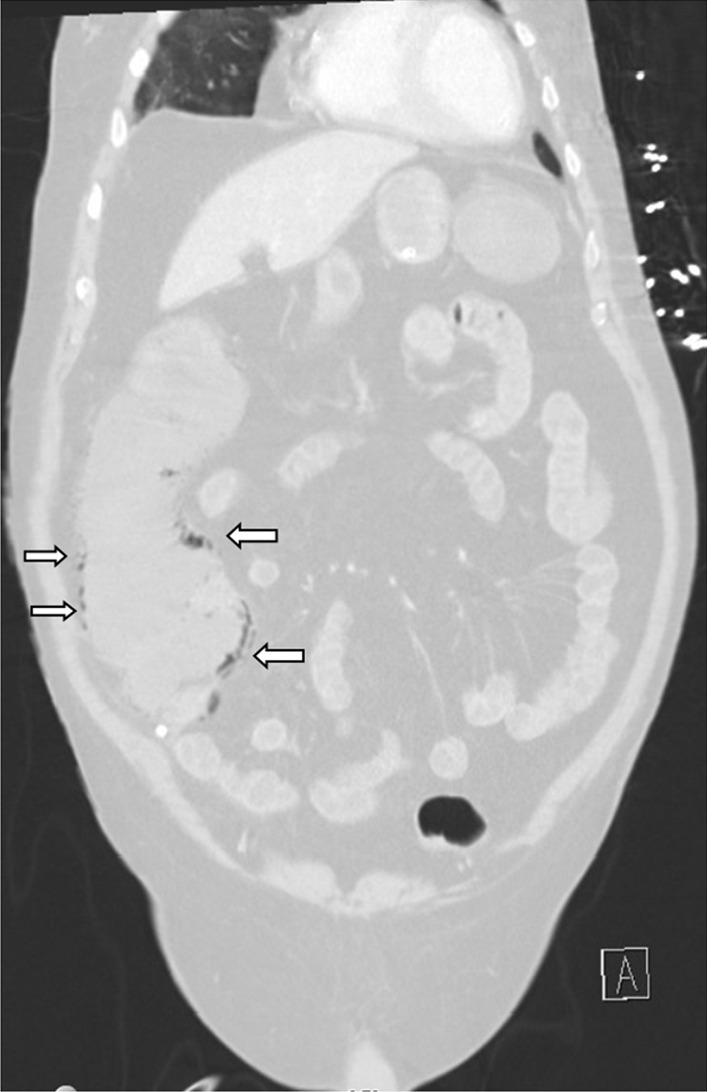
Abdominal computerized tomography (CT) scan with pneumatosis intestinalis of the right colon (arrows) (lung window)
